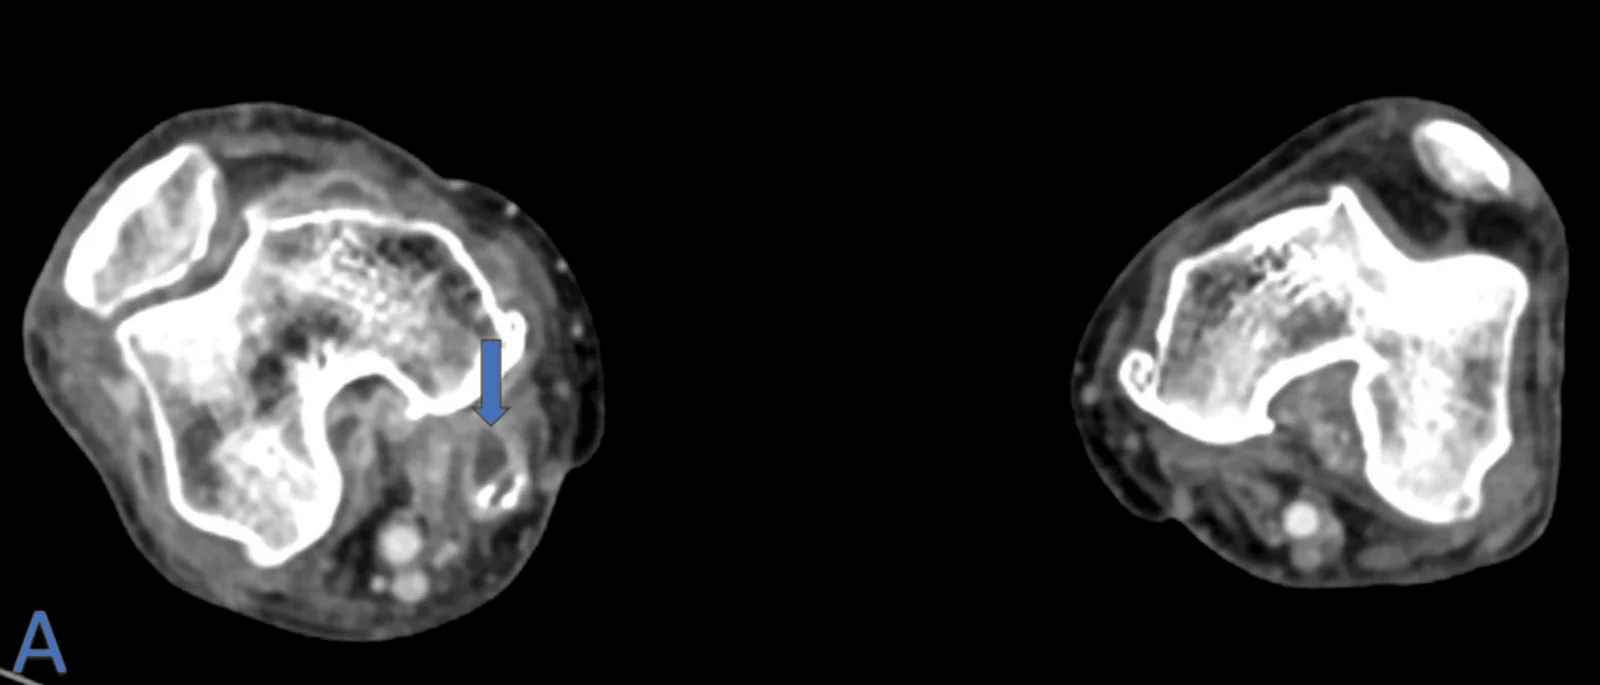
CT images of the knee (A) Axial CT of the knee with the arrow representing an oval contrast-filled structure that matches the contrast attenuation of the adjacent artery at the inferomedial joint space.
